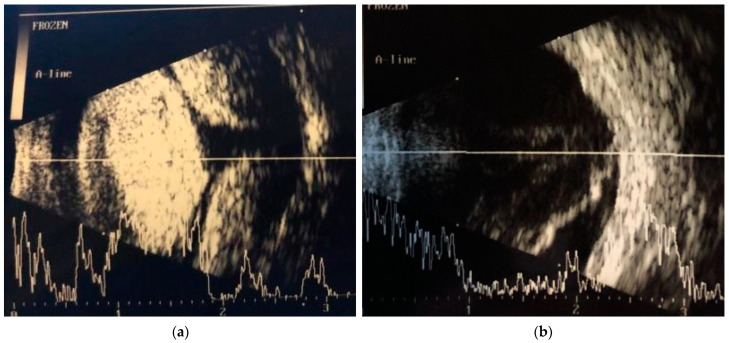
(a) RE echography revealing choroidal detachment 1 day after PKP; (b) RE echography revealing retinal detachment 1 month after PKP. B-scan ocular ultrasound of the RE one day after PRK shows a choroidal detachment, which was treated medically and then surgically drained. However, 1 month after the PKP, she was diagnosed with rhegmatogenous retinal detachment with a horseshoe retinal break inferonasally. Subsequently, she underwent a pars plana vitrectomy 23 Ga with silicone oil, and the retina was reattached. The silicon oil extraction was carried out 2 months after the vitrectomy. Postoperatively, the retina remained attached, but the intraocular pressure (IOP) increased to approximately 30 mmHg under maximal treatment, including systemic carbonic anhydrase inhibitor (CAI). The surgical management of retinal disorders can lead to short-term elevations in intraocular pressure (IOP) and, ultimately, long-term glaucomatous damage if not treated in a timely manner [19]. Elevated IOP is a potentially serious complication following vitreoretinal surgery for a number of reasons. Mechanisms could include secondary pupillary block, preexisting glaucoma [20], chronic inflammation [21], silicone oil migration into the anterior chamber [22], trabecular meshwork obstruction caused by silicone emulsion [23], rubeosis iridis [24], or iridocorneal angle closure caused by anterior synechiae [25,26]. Identifying the mechanism that causes the raised IOP is crucial as the management of glaucoma can differ accordingly [27]. In our case, it was decided that the patient would need another antiglaucomatous surgery, and RE trabeculectomy was performed. Postoperatively, IOP was stabilized in the range of 16–22 mmHg under topical treatment, with fluctuations. Unfortunately, the patient’s condition worsened three months after the trabeculectomy. The patient reported a decrease in visual acuity and pain in the right eye, which began three days before presenting to our clinic. The slit-lamp examination of the anterior segment of the right eye is described below (Figure 3). The left eye presented with a clear cornea, oval pupil, deep anterior chamber, and aphakia. IOP was 30 mmHg in RE and 12 mmHg in LE in both eyes with maximal treatment: topical CAI twice daily (BID), fixed combination beta-blocker and prostaglandin analog once daily (QD), and systemic CAI QD.
